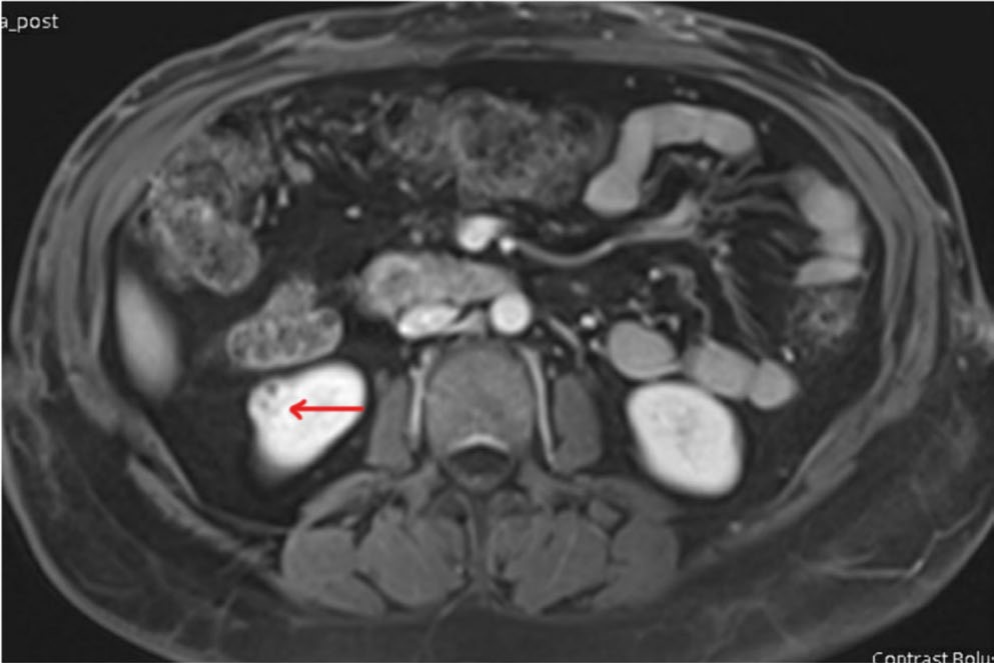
MRI scan of the abdomen with contrast showing right renal mass suggestive of renal cell carcinoma.
